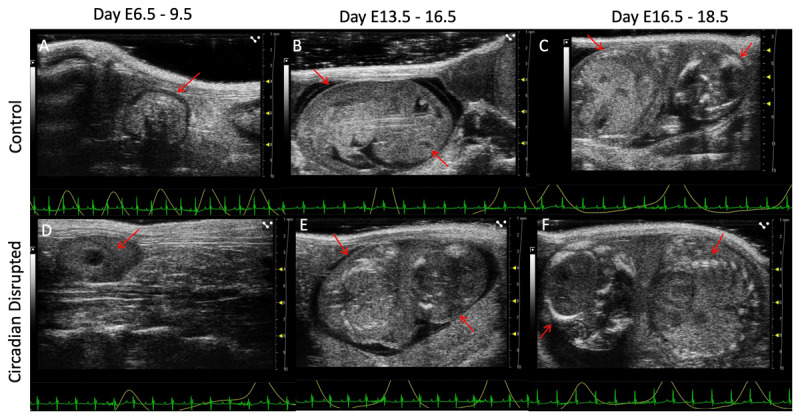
Representative images of high frequency ultrasound images. A) Control implantation site at day 8.5. indicated with red arrows; B) Control fetus at day 14.5; C) Control fetus at day 16.5. Notice the calcified vertebrae and eye indicated with red arrow; D) Circadian disrupted implantation site at day 6.5 indicated with red arrow; E) Circadian disrupted fetus at day 14.5. F) Circadian disrupted fetus at day 17.5 with calcified skull and vertebrae indicated with red arrow.
